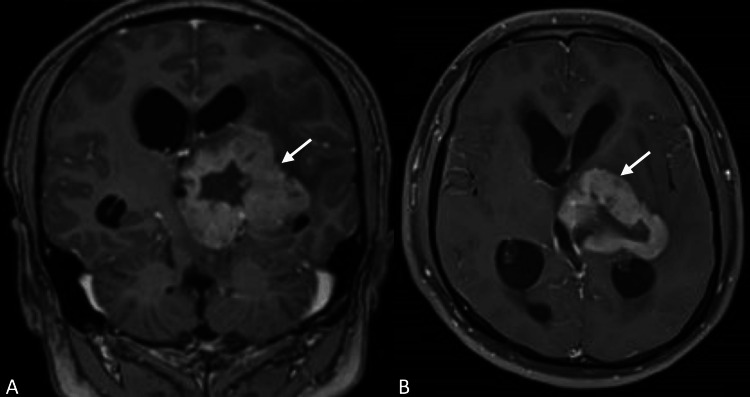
Preoperative T1-weighted contrast MRI (A) Coronal and (B) axial views with enhancing tumor at the left thalamus (arrows) MRI, magnetic resonance imaging
